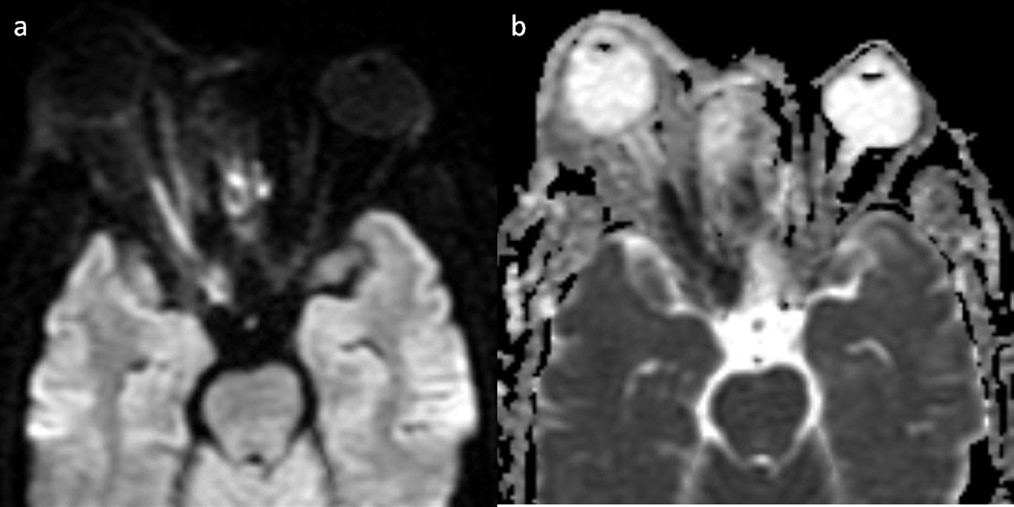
Axial DWI (a) and ADC (b) images showing optic nerve infarction.
